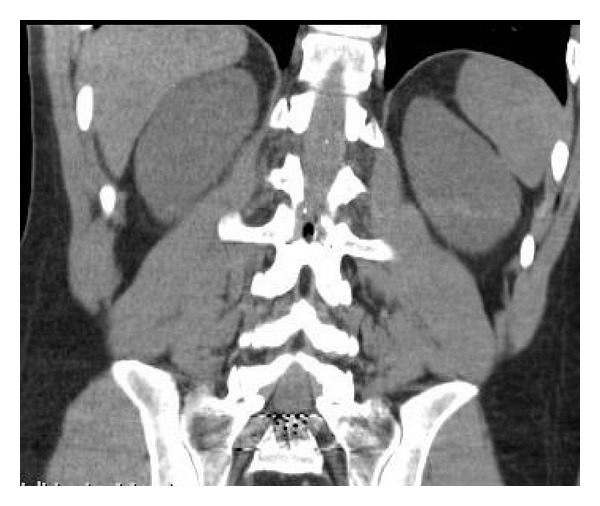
In the coronal CT scan we can see the damage to the posterior arc of L2 that was the entry point of the bullet.
